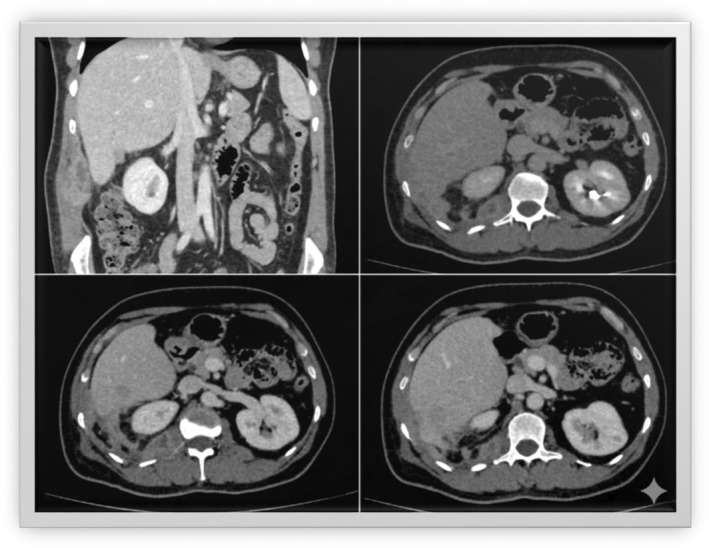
Contrast‐enhanced computed tomography (CT) of the abdomen at presentation demonstrating two hypodense lesions within the right hepatic lobe, consistent with developing liver abscesses. Coronal (upper left) and axial (upper right, lower left, and lower right) images show irregular low‐attenuation lesions with surrounding inflammatory changes. Serial follow‐up imaging demonstrated only partial interval regression despite culture‐directed antibiotic therapy, prompting further diagnostic evaluation for an atypical infection.
